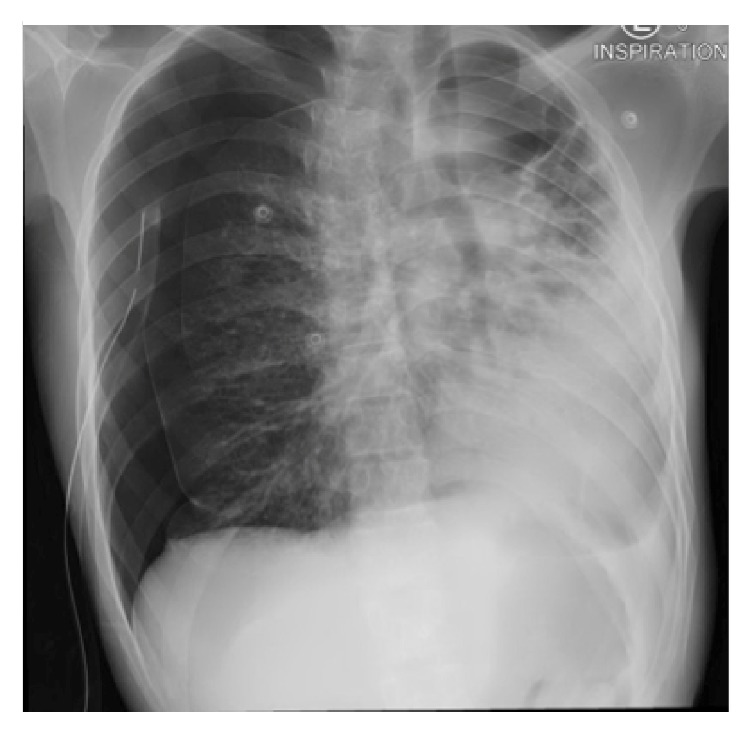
Chest X-ray showing worsening of the pneumothorax despite presence of a chest tube.
